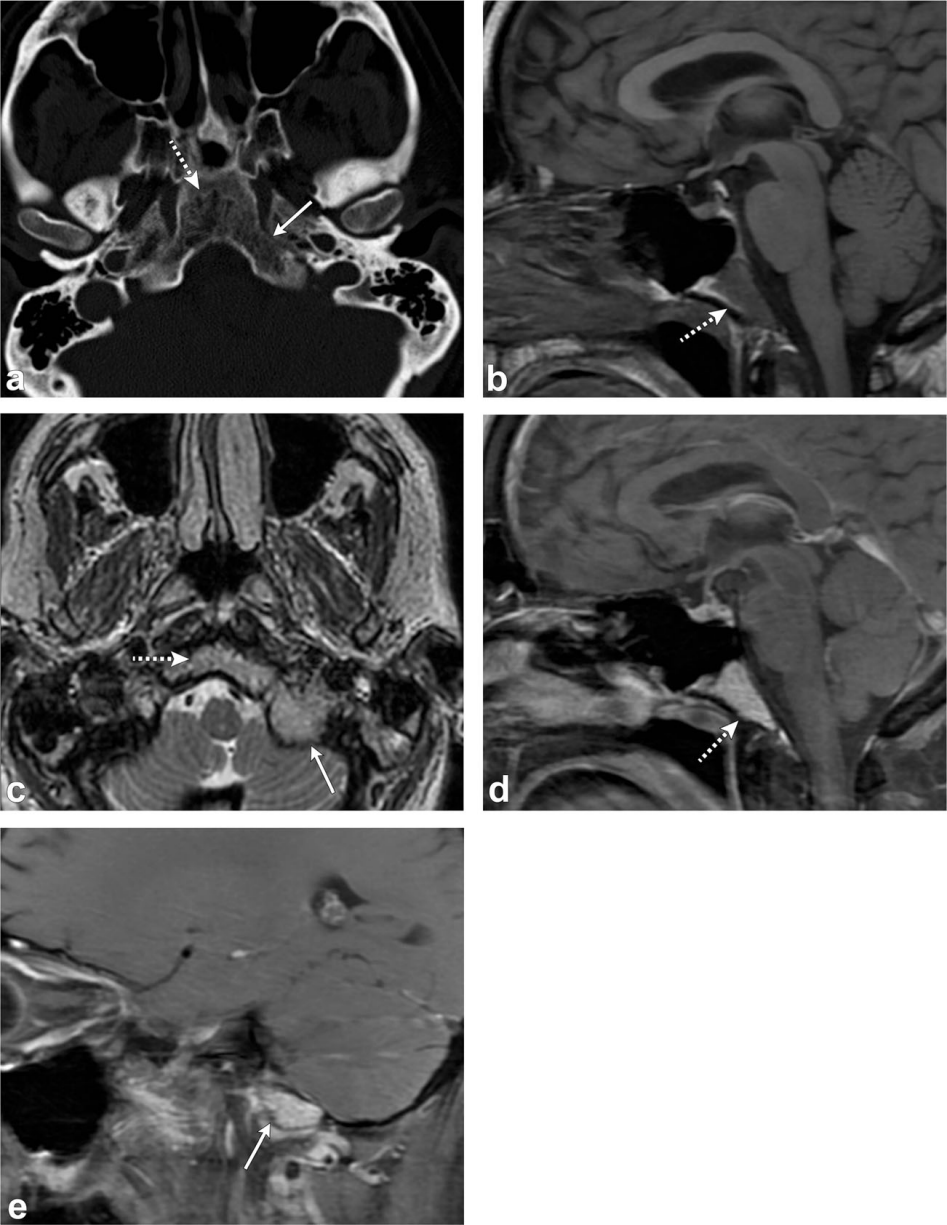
Osseous venous vascular malformation. Axial head CT (a) shows osteolytic changes (trabecular appearance) of the clivus (*dashed arrow*) extending into the left occipital condyle (*thin arrow*). Sagittal T1-weighted (b), axial T2-weighted (c) and post-contrast sagittal T1-weighted images (d, e) show abnormal signal in the clivus (*dashed arrows*) and left occipital condyle (*thin arrow*) with enhancement
